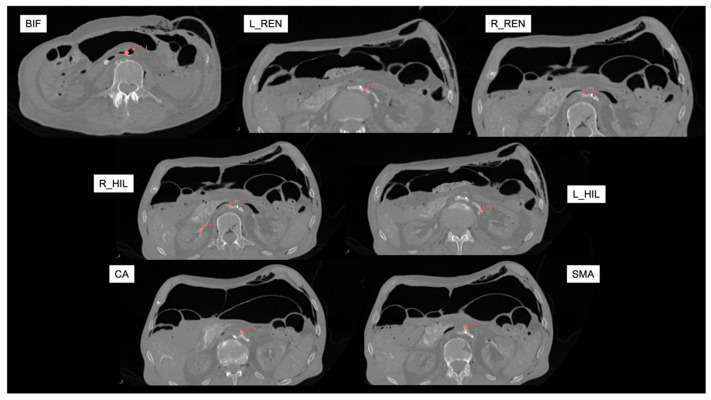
Reference axial CT angiography images (in supine position with zero mmHg intra-abdominal pressure) for marking targets. Red virtual fiducials were manually placed to mark each target landmark. BIF: aortic bifurcation; L_REN: left renal artery; R_REN: right renal artery; L_HIL: left hilum; R_HIL: right hilum; CA: Celiac artery; SMA: Superior Mesenteric artery.
